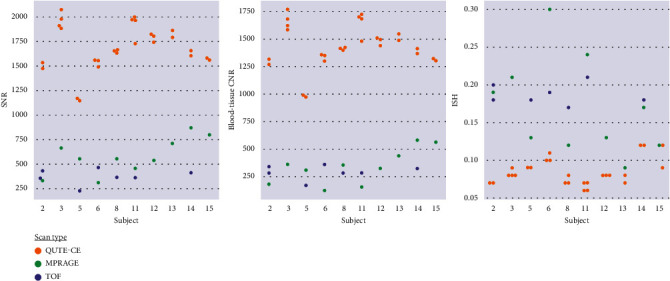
Signal-to-noise ratio (SNR), intraluminal signal heterogeneity (ISH), and contrast-to-noise ratio (CNR) for participants with directly comparable scans. Quantitative Ultrashort Time-to-Echo Contrast-Enhanced MR angiography (QUTE-CE MRA) provides higher blood SNR and blood-tissue CNR than the time-of-flight (TOF) MRA or T1-weighted ferumoxytol contrast-enhanced Magnetization Prepared RApid Gradient Echo (Fe MPRAGE) in every participant while maintaining lower ISH. Participants are excluded in cases where scans were significantly motion-degraded.
